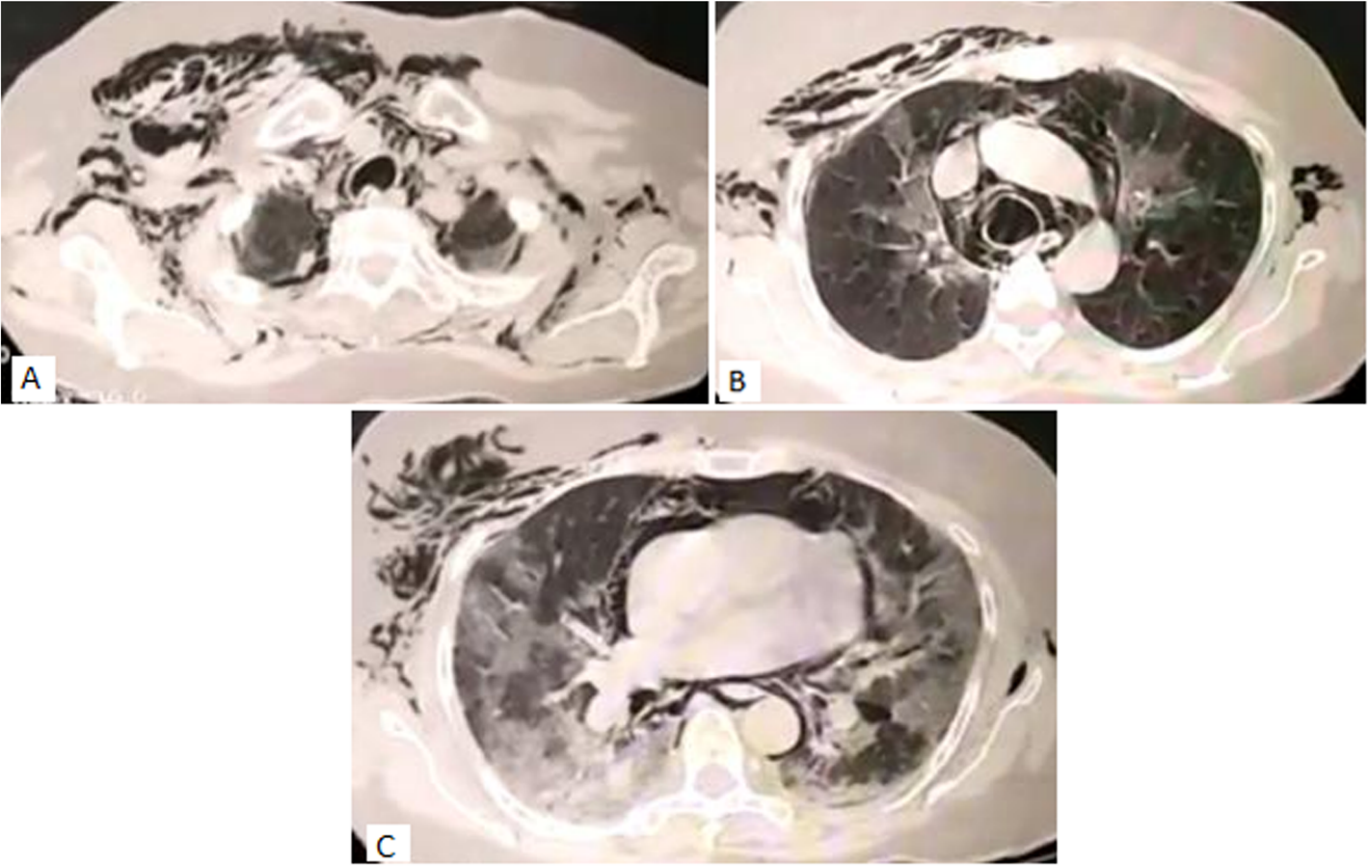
Axial CT chest images showing. A Subcutaneous emphysema extending up to posterior chest wall. B Presence of air around the trachea and major blood vessels indicative of pneumoediastinum along with bilateral upper lobe ground glass opacities. C Presence of air dissecting the pericardium indicative of pneumopericardium with bilateral extensive lower lobe ground glass opacities
